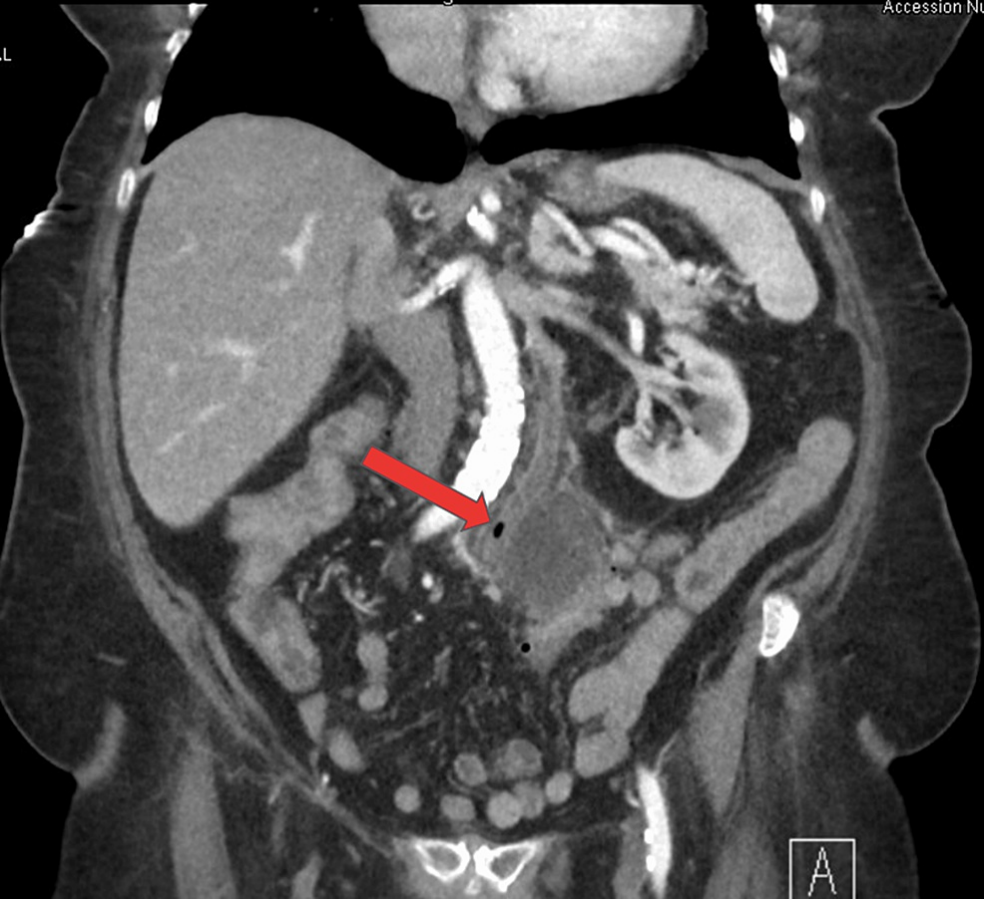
Initial computed tomography of the abdomen showing diverticular abscess and superior mesenteric vein thrombosis
